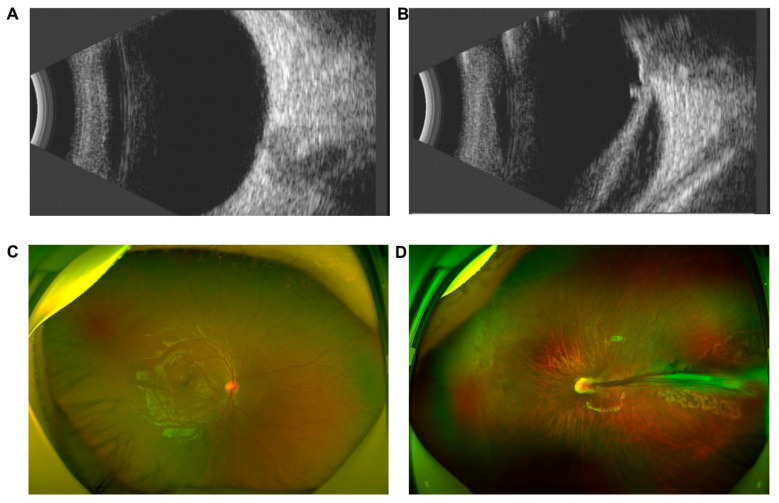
Clinical features of DX1561. B-scan ultrasonography showed a normal retina and vitreous in the right eye (A) and retinal folds in the left eye (B). Scanning laser ophthalmoscopy showed a normal retinal anatomy of the right eye (C) and retinal folds in the left eye (D).
